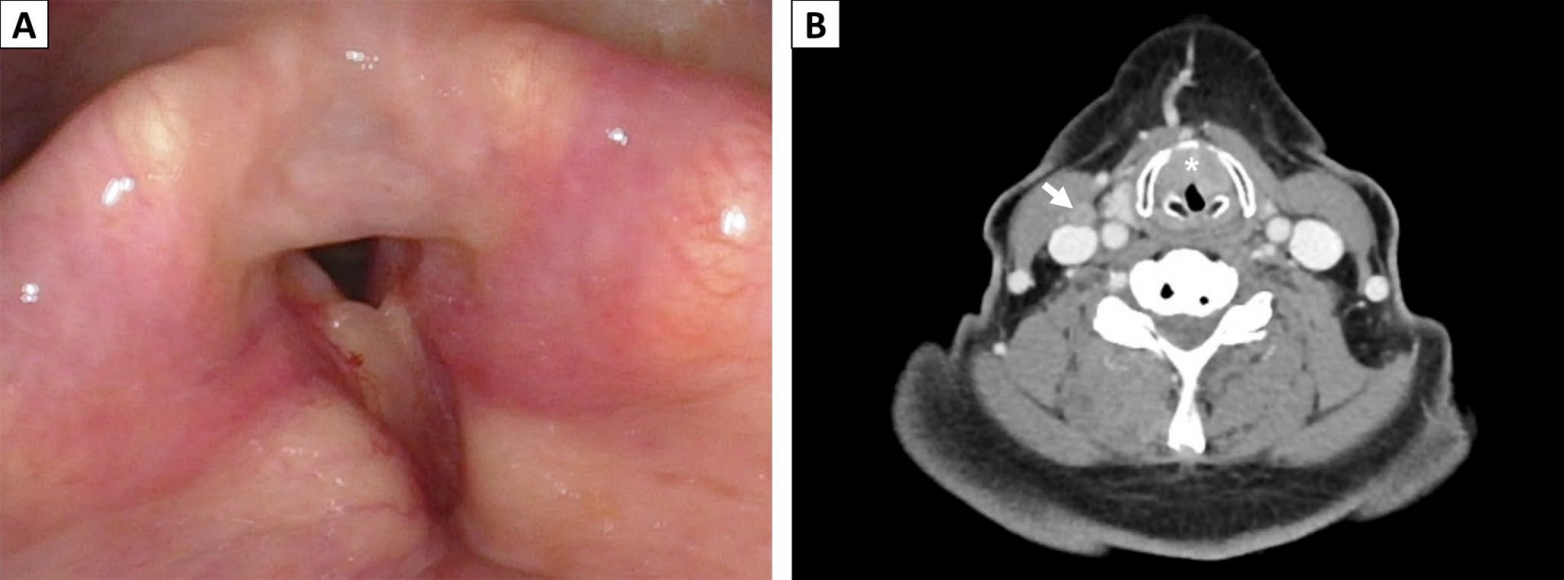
Clinical presentation. (A) Nasopharyngoscopy revealed adhesion of the anterior two-thirds part of the true cords. (B) Neck computed tomography showed increased soft tissue components involving the anterior commissure (asterisk) and the anterior part of bilateral vocal cords. One enlarged lymph node on right level III was highlighted (arrow)
